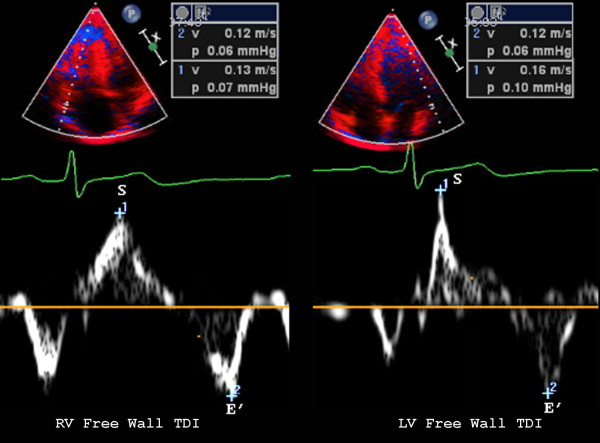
Examples of color Doppler tissue imaging lateral to the tricuspid valve (left) and mitral valve (right) from the four chamber view including peak systolic (S) and diastolic (E') velocities.
